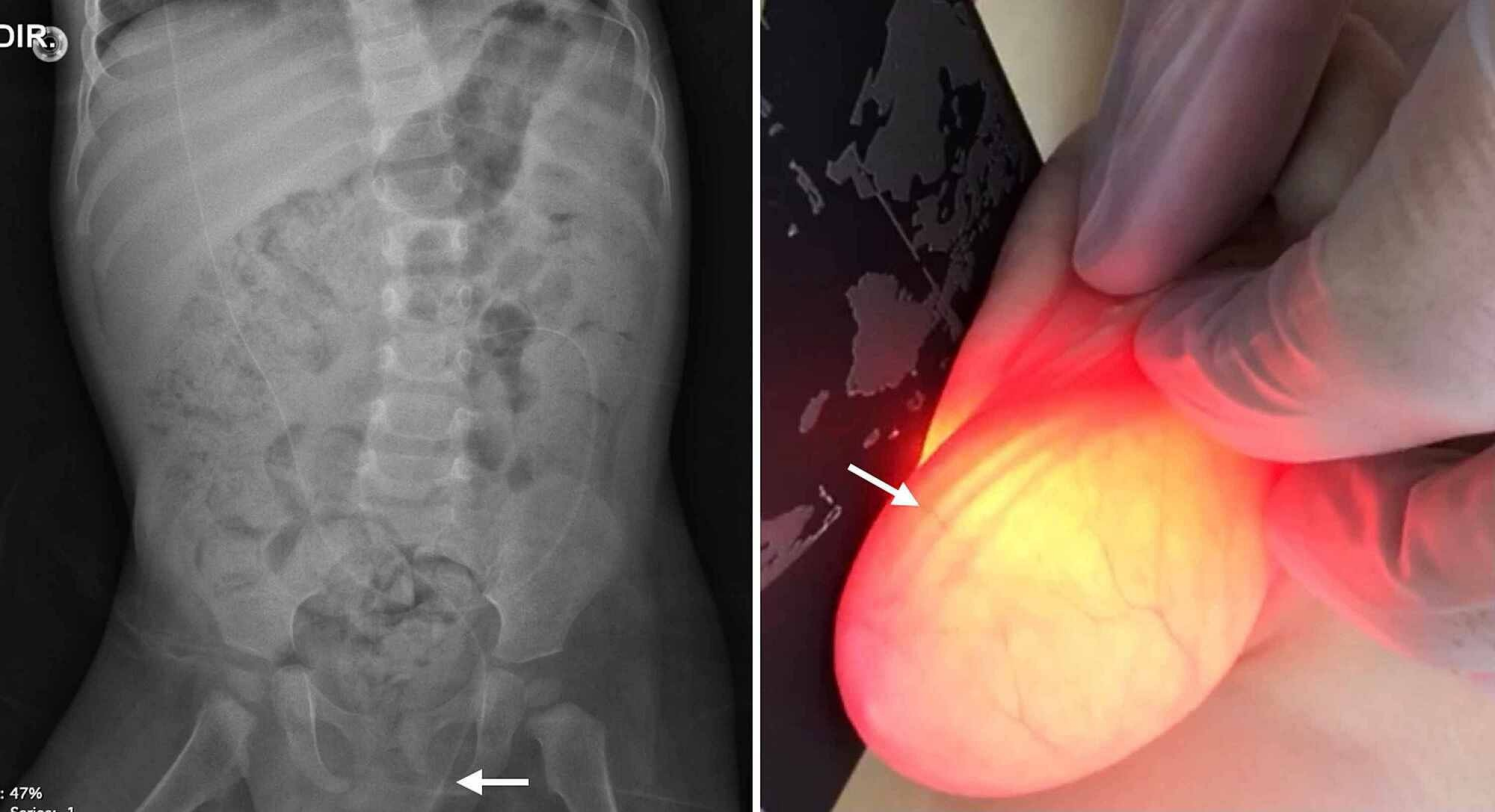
Migration of distal VPS catheter to scrotum. Abdominal X-ray shows the migration of distal catheter of VPS (white arrow) to the left scrotum (left). During the physical exam, a transillumination of the left scrotum allows to perceive the entanglement of distal catheter inside of this region (right). VPS: ventriculoperitoneal shunt.
